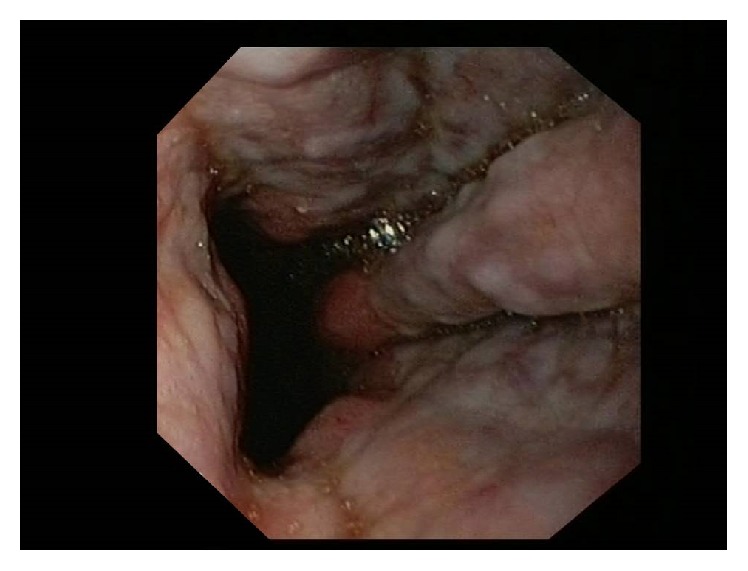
Grade III esophageal varices visualized by endoscopic ultrasound technique.
